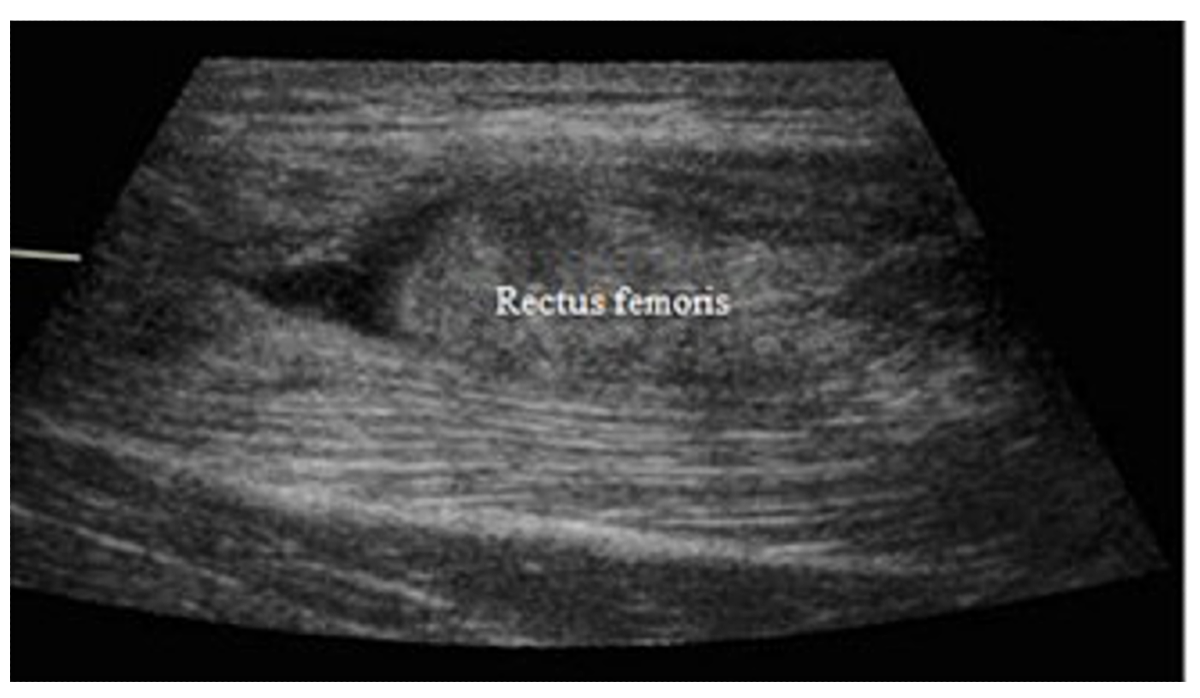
Rectus femoris ultrasound imaging.
